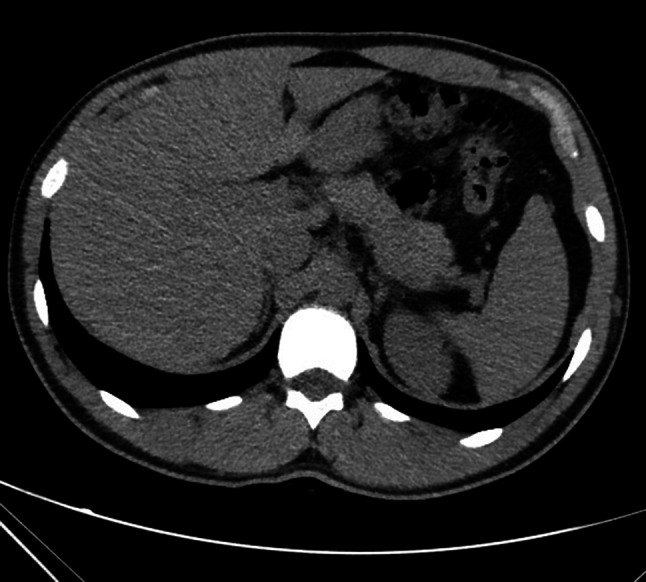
Native low-dose CT demonstrated no sign of aerobilia or interstitial air 60 min after contrast injection
